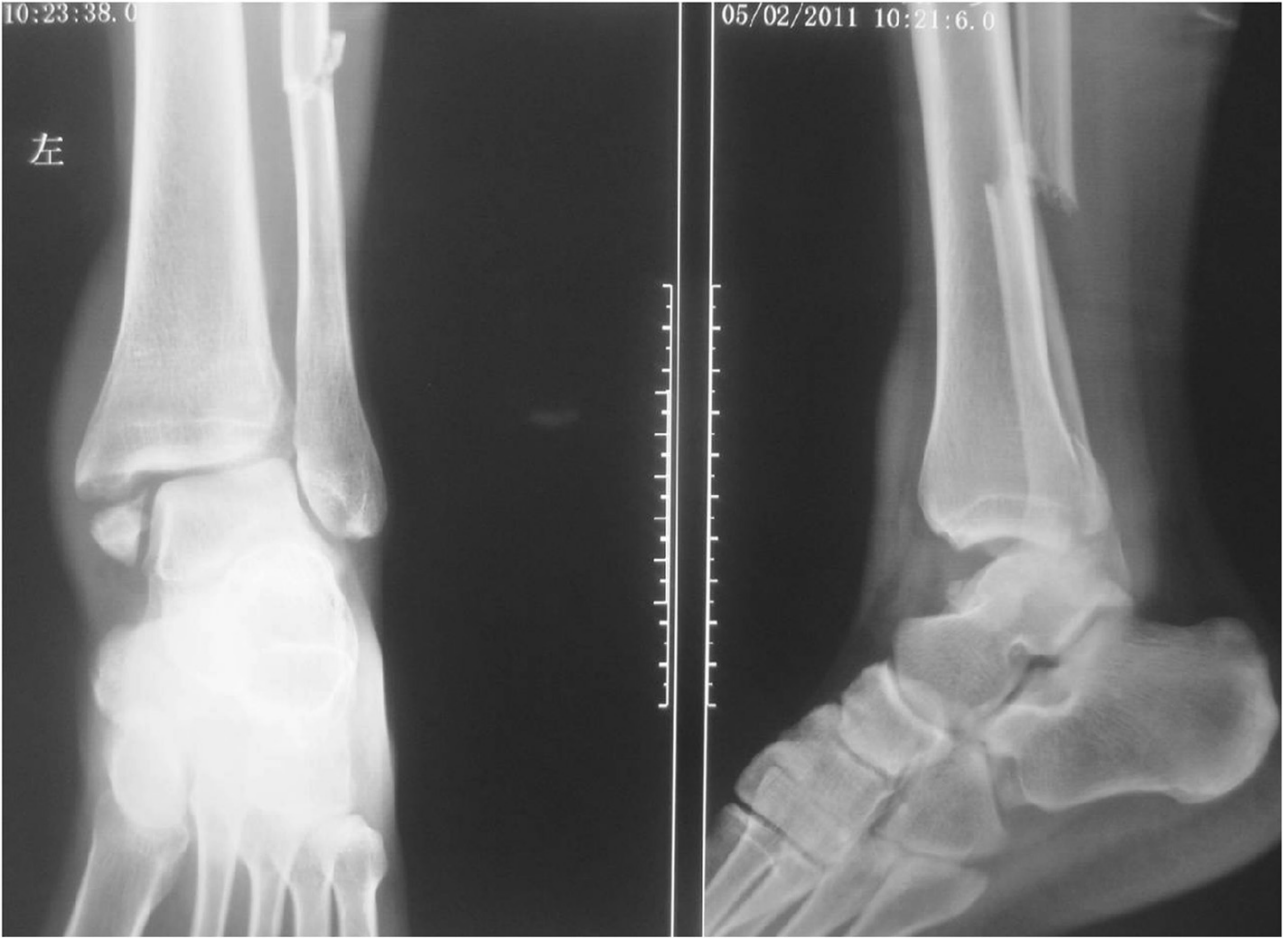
The pre-operation radiograph shows that the fracture type was 44C23 by AO classification combined with distal tibiofibular diastasis.
